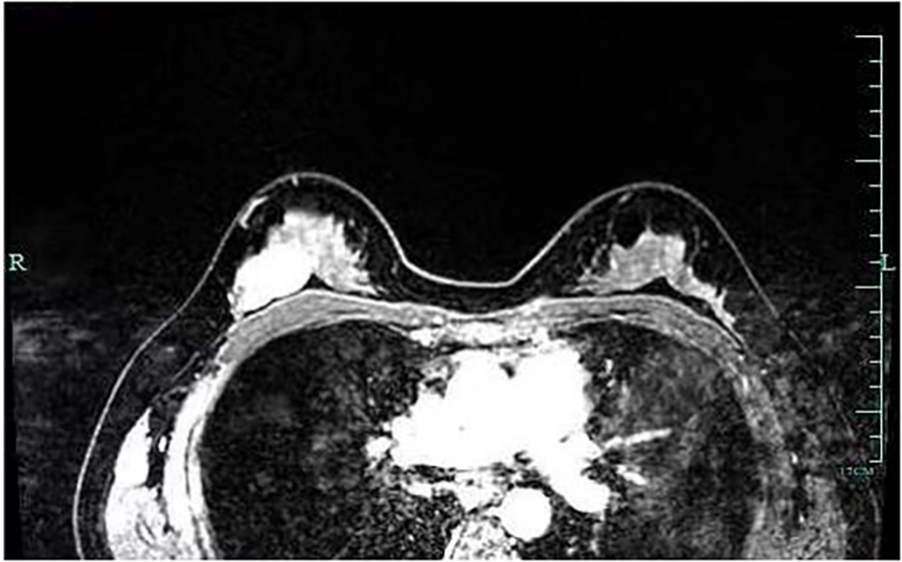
Breast MRI indicated a nodular lesion of abnormal enhancement, with clear boundary and slightly irregular shape.
